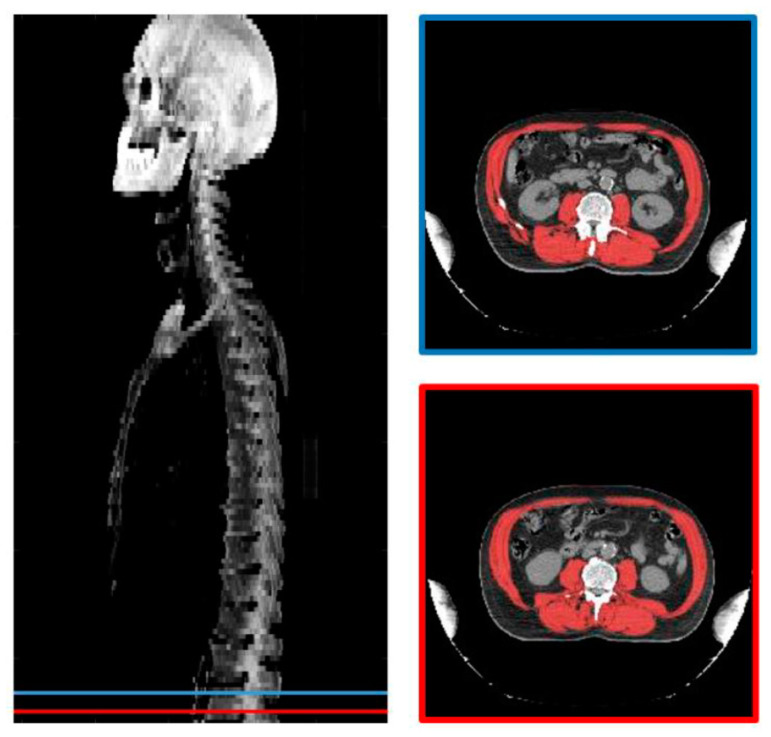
(Left) coronal (Maximum Intensity Projection) MIP centered on the vertebral column and the 2 slices selected at the L3 level; (right) axial CT slices selected and the segmentation of the skeletal muscles.
